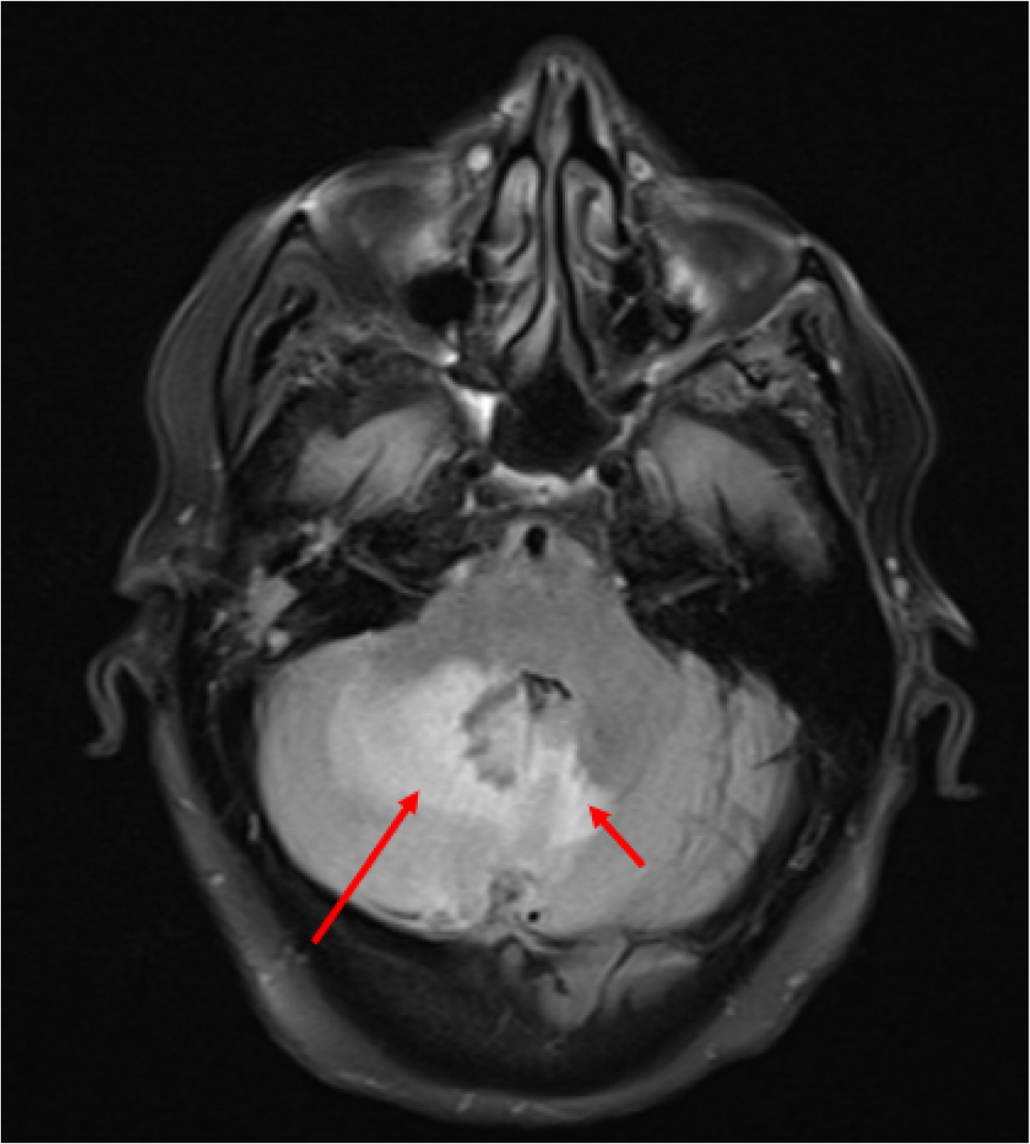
Axial T2 FLAIR sequence MRI at the level of the 4th ventricle within the posterior fossa demonstrating diffuse right cerebellar hyperintensity (long red arrow) and to a lesser extent left cerebellar hemisphere hyperintensity (short red arrow) corresponding to the large amount of vasogenic edema produced by the lesion
